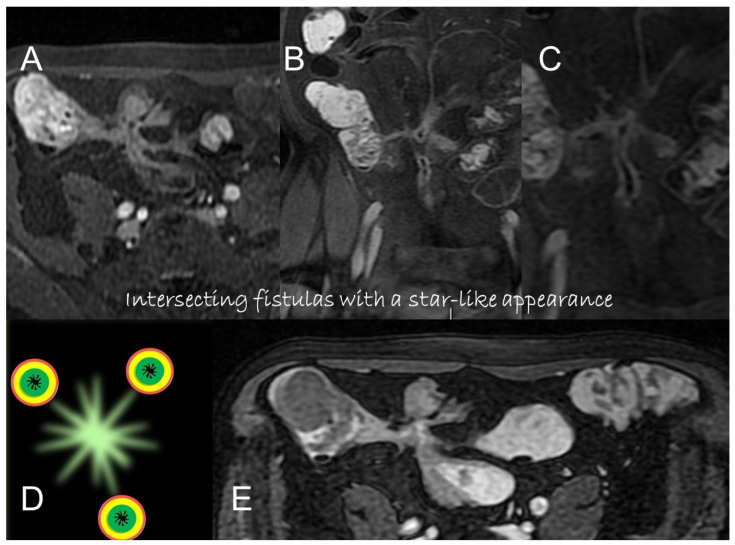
Axial (A) and coronal (B,C) contrast-enhanced fat-suppressed T1-weighted images and axial FIESTA image (E) of the same patient show a complex fistula formed by the intersection of multiple fistulous arms that connect pathological segments of the small intestine to each other and to the ascending colon through the mesentery. These fistulous pathways, intersecting each other, end up taking on the starry appearance, typical of complex fistulas (D).
